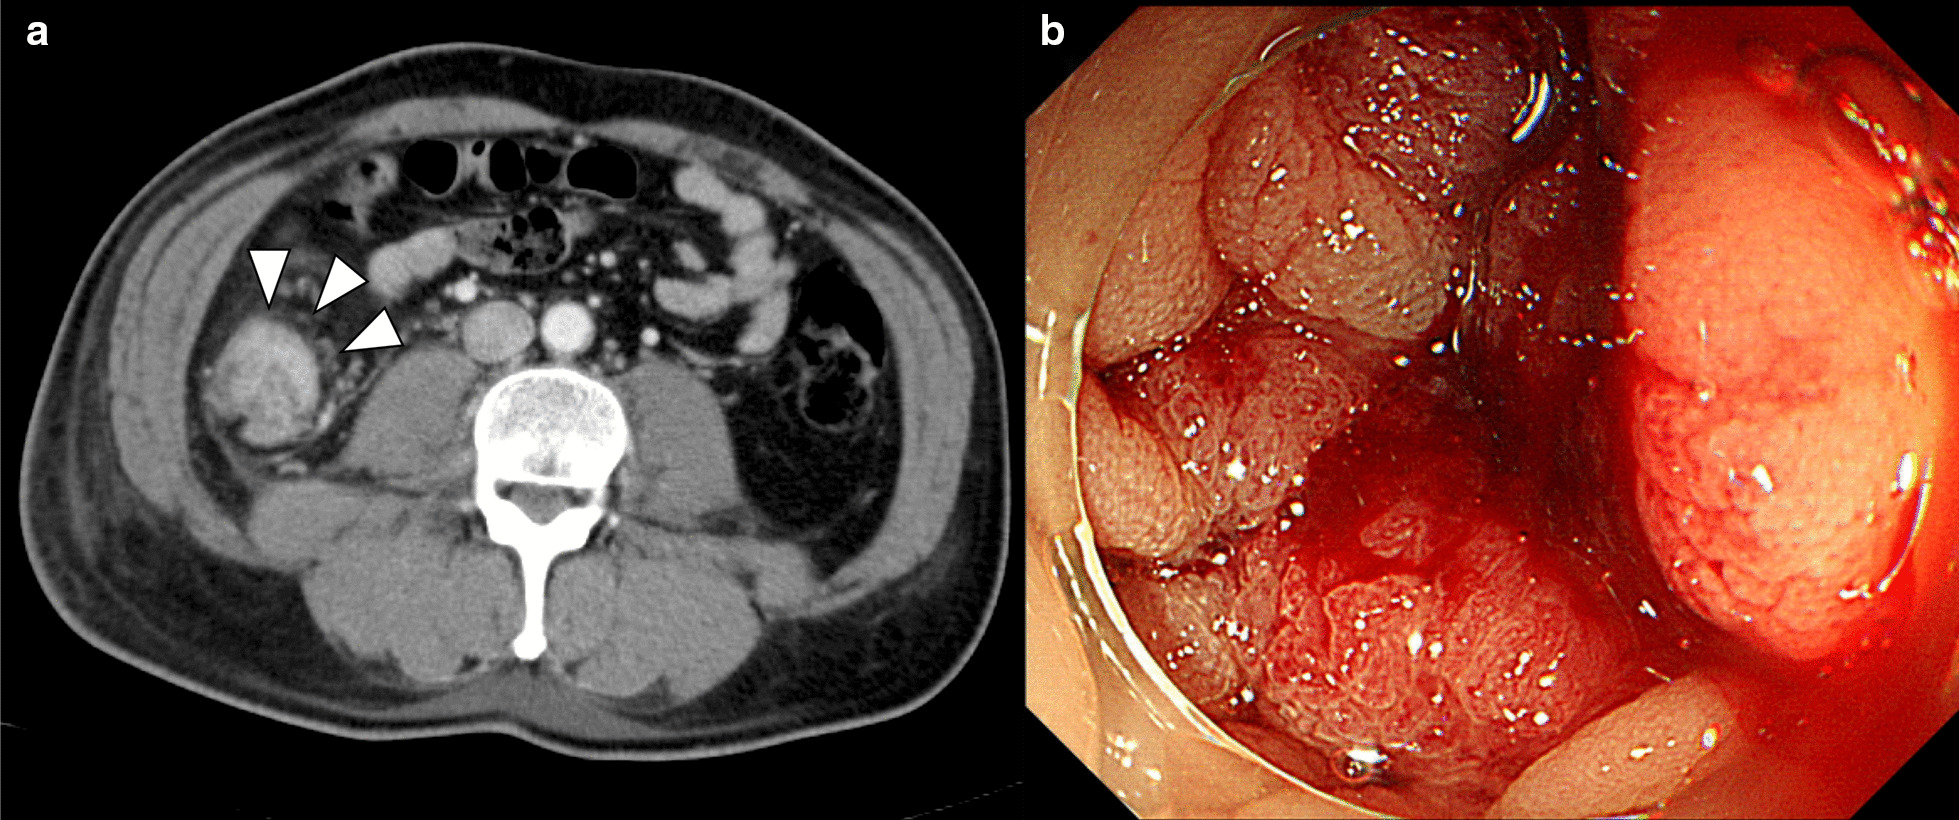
Colonoscopy revealing the ascending colon tumor. Contrast computed tomography showing a tumor mass in the ascending colon (white arrows) with no other notable metastases (a), and colonoscopy revealing an ascending colon tumor (b)
